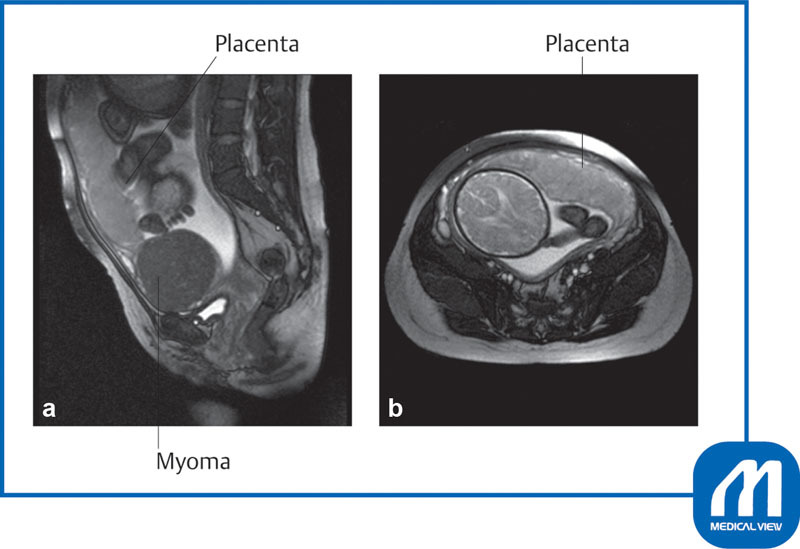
Case 3: myoma on anterior cervix magnetic resonance imaging findings (T2W). This patient had an 11-cm uterine myoma on the anterior wall of the cervix, which was in close contact with the placenta on the anterior uterine wall and extended to 11 cm above the umbilicus.
